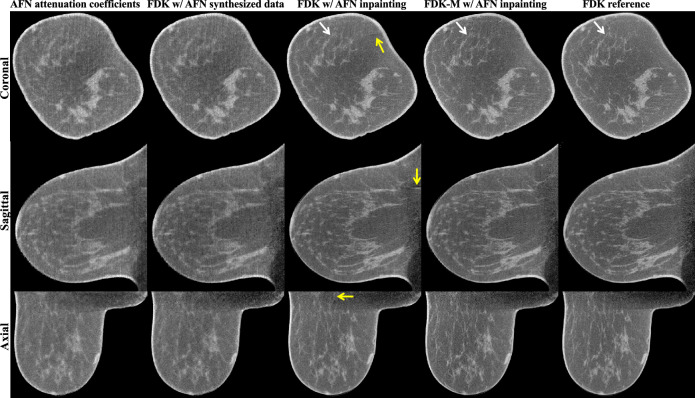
Breast CT image reconstructions (11.8 cm chest-wall diameter) with AFN and/or FDK using incomplete data (columns 1–4) are compared to the FDK reference using complete data (column 5). A trained AFN can either directly output the image volume (column 1) or synthesize projection data for subsequent reconstructions (columns 2–4). When FDK uses the AFN synthesized complete data (column 2), it produces images visually similar to the “AFN attenuation coefficients”, showing a loss of resolution. When the acquired projection data are reused and spliced with AFN synthesized data (column 3), a subsequent FDK reconstruction recovers the lost resolution yet exhibits truncation-like artifacts (yellow arrows) due to the slight inconsistency between AFN synthesized projections and the acquired projections. We incorporated an offset-detector weight into the FDK algorithm, denoted as FDK-M. This weighted FDK reconstruction using AFN inpainted data (column 4) effectively eliminates the line artifacts. The display window is [0.15, 0.35] cm^−1^.
